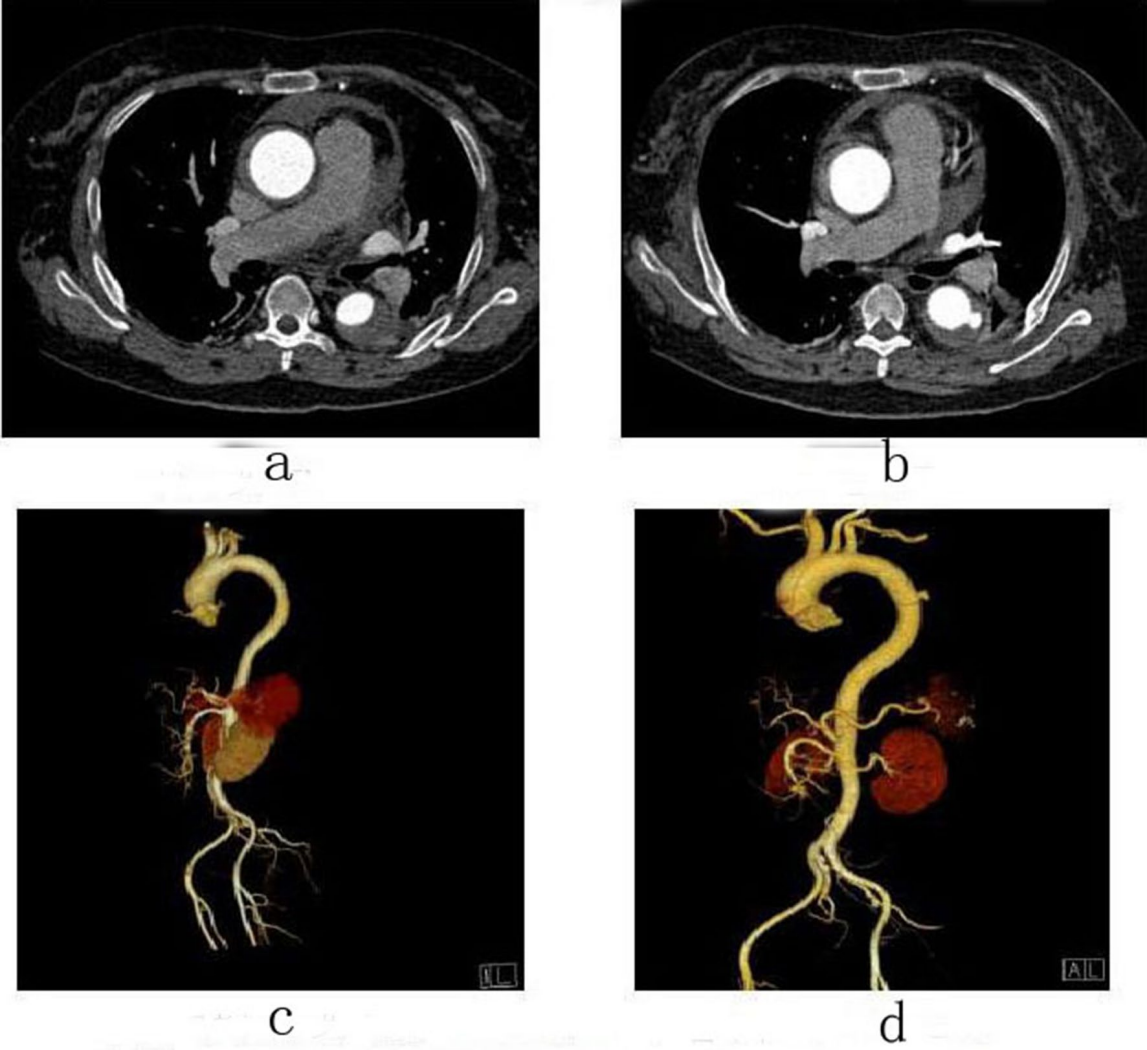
Female, 57 years old, admitted to hospital for “sudden pain in low back for 15 h”, Diagnosis: Stanford type A aortic dissection; treatment: IMH conservative treatment. CTA of the patient in different periods: (a) Admission; (b) 12 days after admission; (c) Admission; (d) 12 days after admission.
